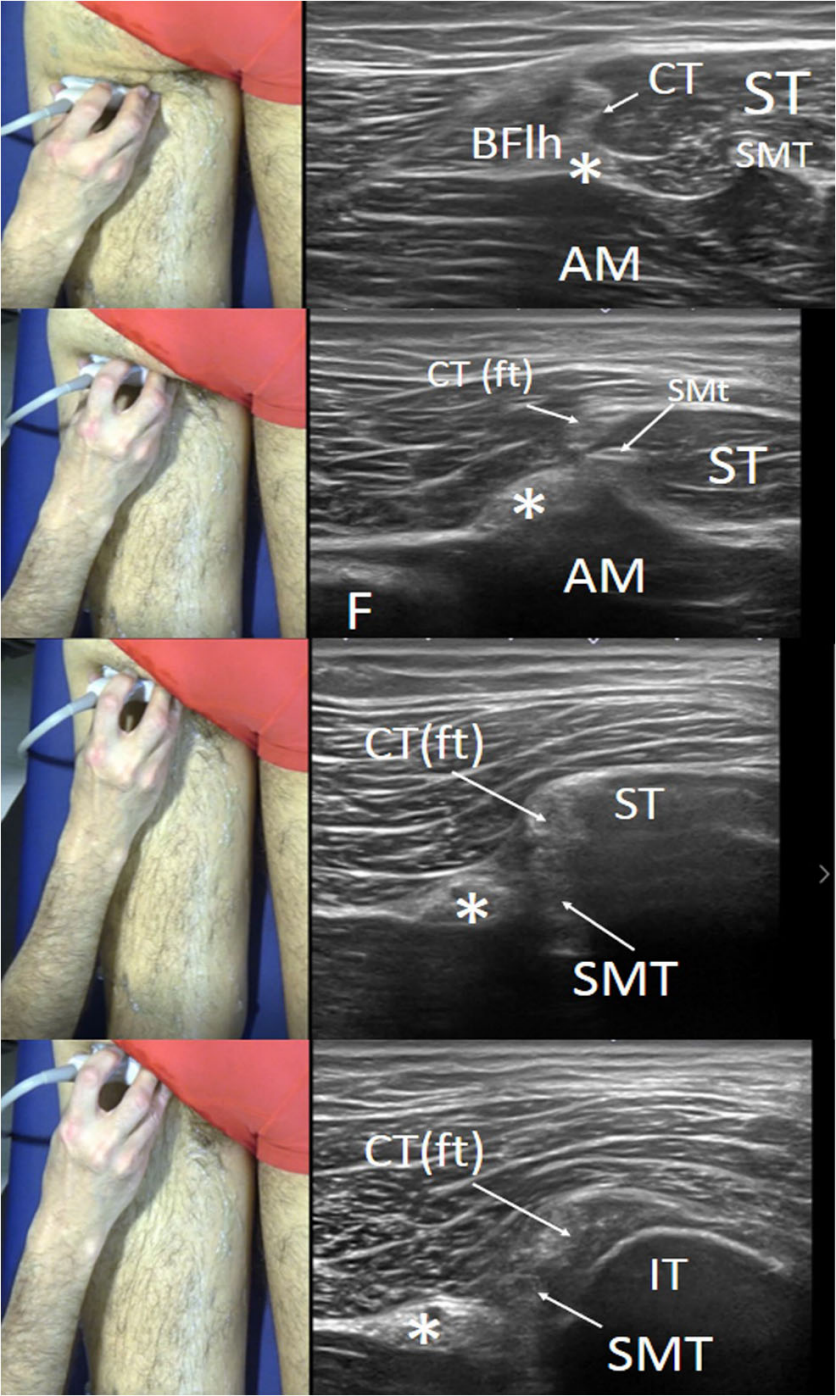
Series of short-axis ultrasound scans showing different views of the proximal part of the hamstrings (handle of the tuning fork). As the probe is moved in a proximal direction, the sections of the common tendon and the semimembranosus tendon can be seen approaching the sciatic nerve until a hyperechoic line is observed with a posterior acoustic shadow consistent with the ischial tuberosity. *BFlh* long head of the biceps femoris, *ST* semitendinosus muscle, *AM* adductor magnus muscle, *SMT* semimembranosus tendon, *CT* common tendon, *CT (ft)* free-tendon part of the common tendon, (*) sciatic nerve. The photographs on the left of the figure indicate probe positioning
